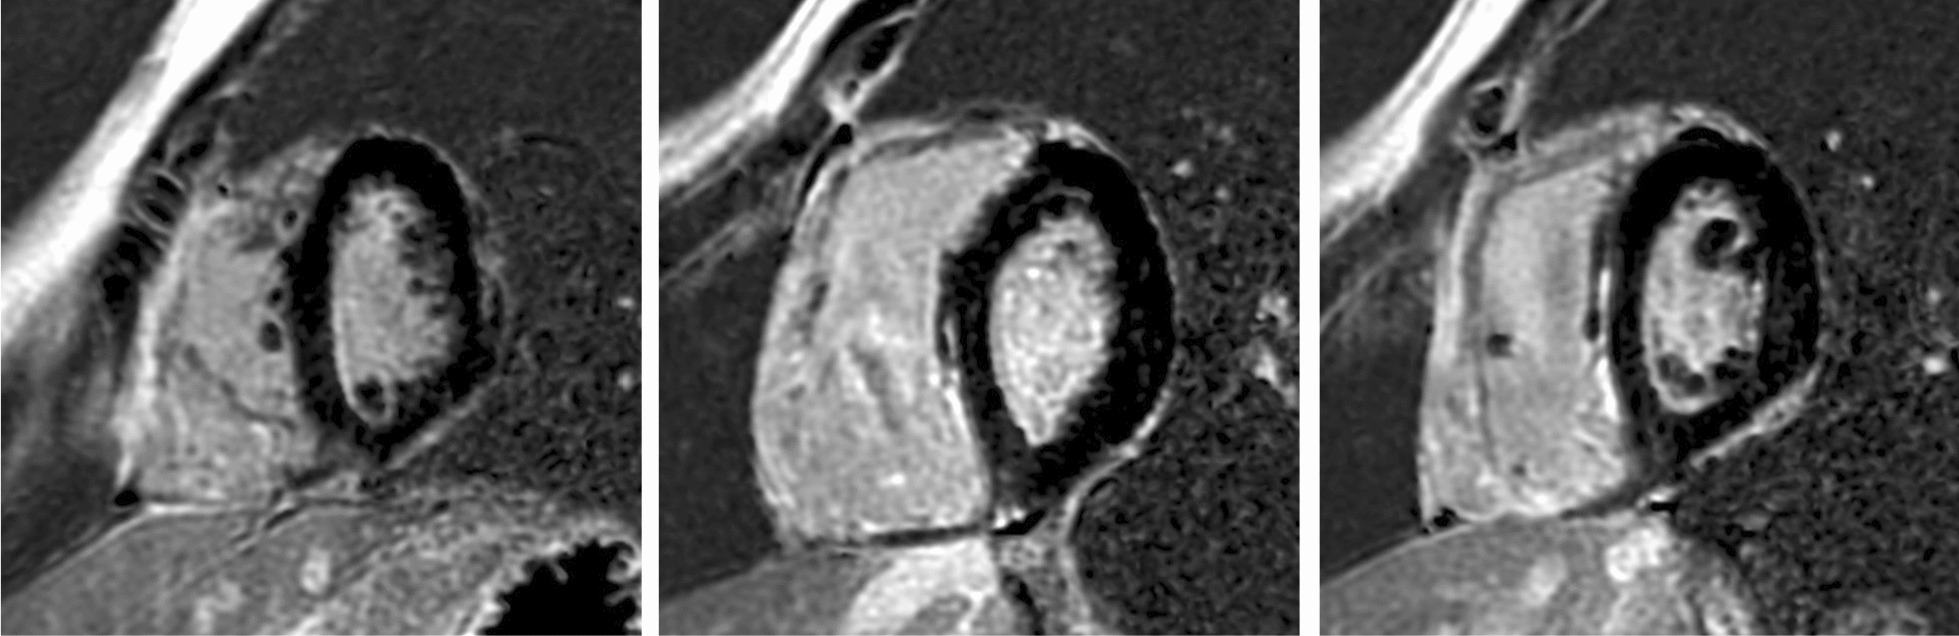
Cardiac Magnetic Resonance Imaging showed intramyocardial late gadolinium enhancement due to diffused fibrofatty scar involving the RV free wall
